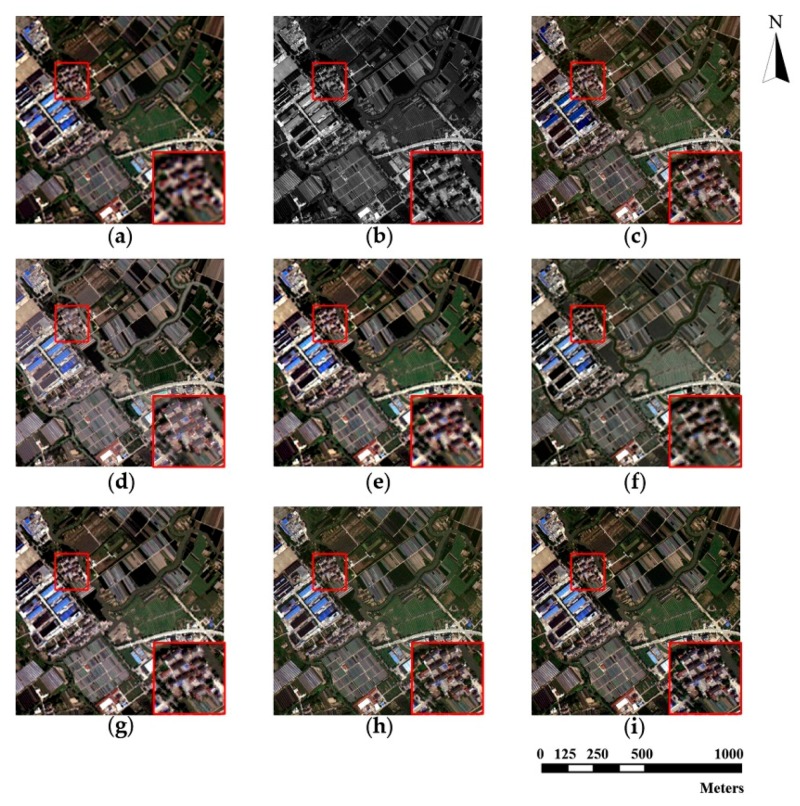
Triplesat original images and fusion results of different methods. (a) MS; (b) PAN; (c) GS; (d) AIHS; (e) WT-SR; (f) WT-IHS; (g) CT-ICA; (h) CSI; (i) proposed.
